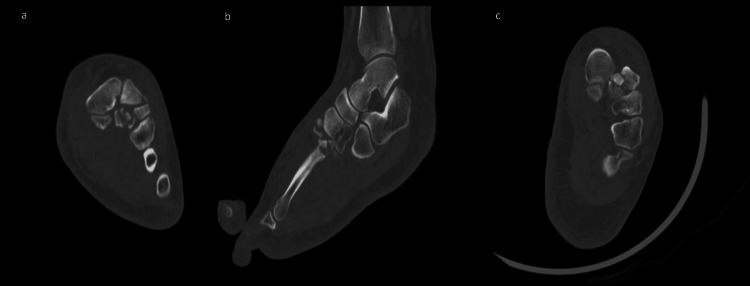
CT images of the left foot (a: axial, b: sagittal, c: coronal) demonstrating a comminuted intra-articular fracture at the base of the second metatarsal with widening of the first-second intermetatarsal space, confirming an unstable Lisfranc fracture-dislocation.
